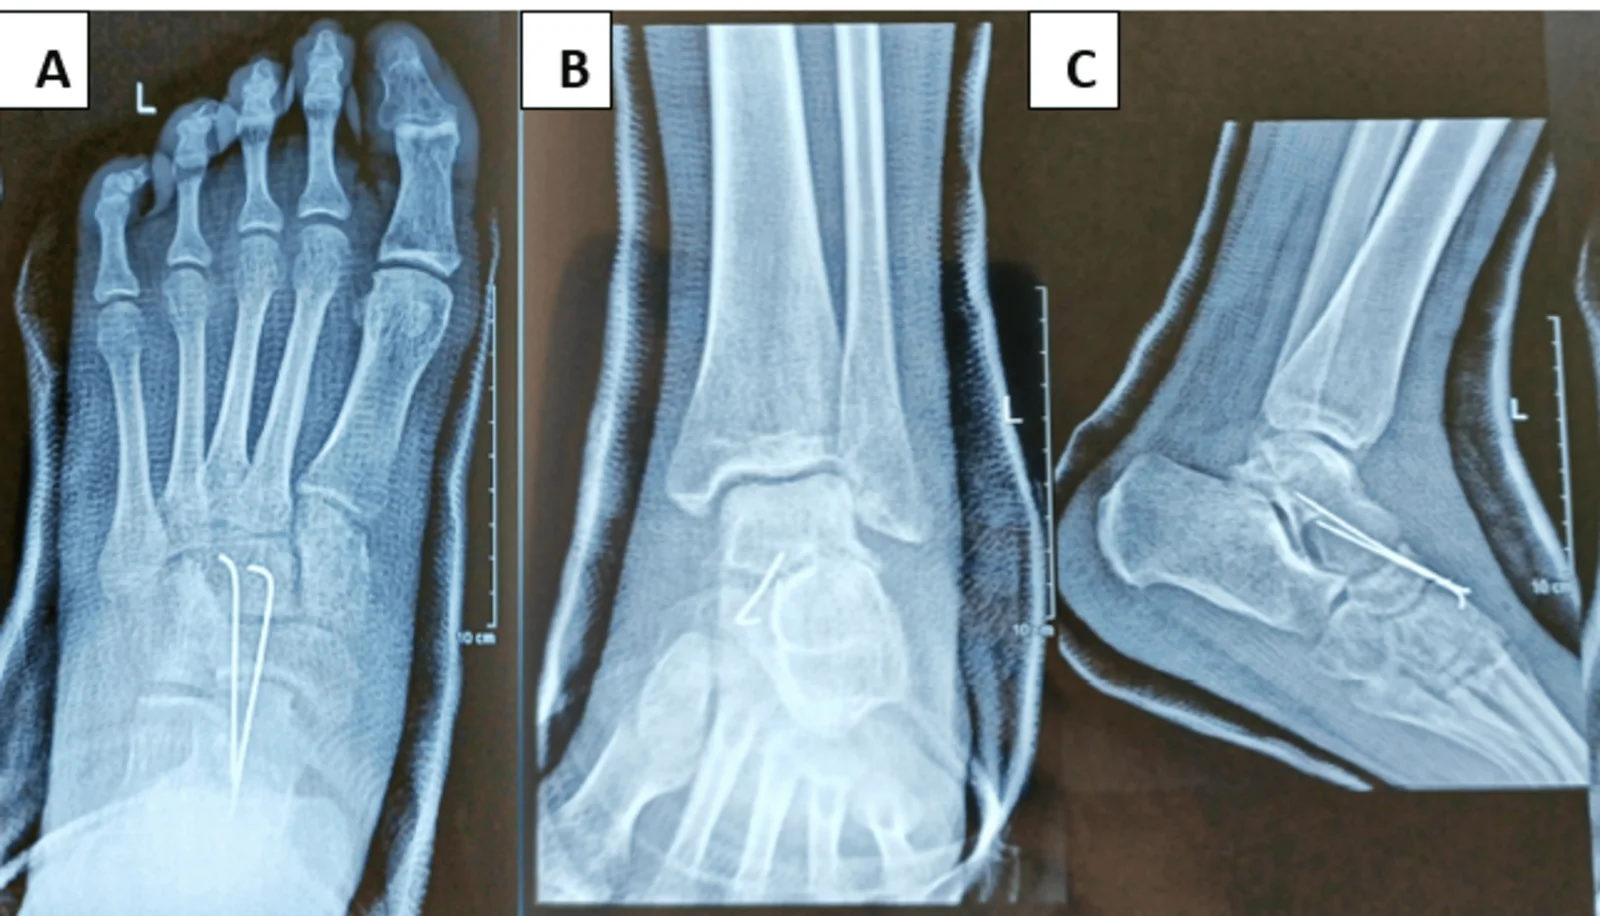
Postoperative radiographs two weeks after surgery (A and B) Anteroposterior view and (C) lateral view
